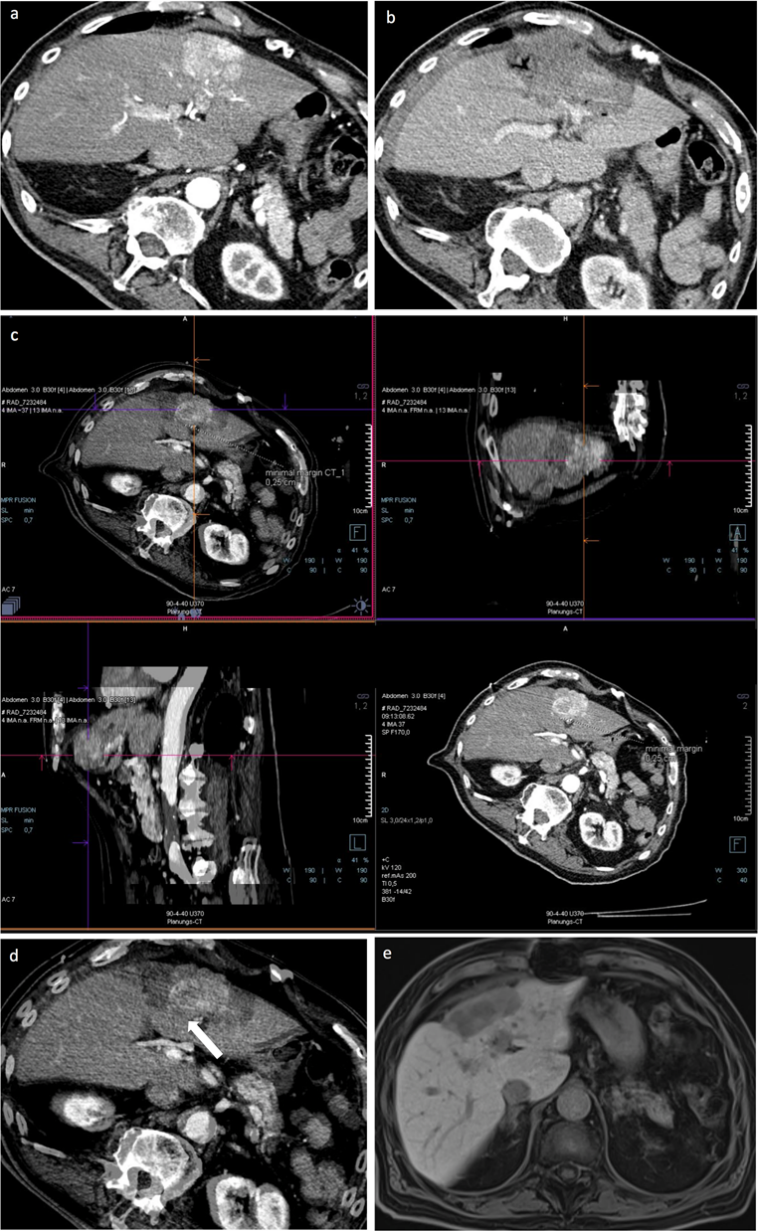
An 88-year-old male patient treated with stereotactic RFA. a The arterial phase of pre-SRFA CT scan of a single HCC lesion with a maximal diameter of 6 cm in liver segments II, III, IVa, and IVb. b The late portal venous phase of post-SRFA CT scan showing clear demarcation of the ablation zone. c The after fusion of pre- and post-SRFA CT scan with manual registration by multiplanar slight shifting (translation and rotation) in axial, coronal, and sagittal planes referring to intrahepatic structures such as vessel bifurcation. d The axial plane of CT image fusion with a MAM of 2.5 mm in clock position 6–8 h (arrow). e MRI scan 24.9 months after ablation without evidence of LTP and progressively shrinking ablation zone
